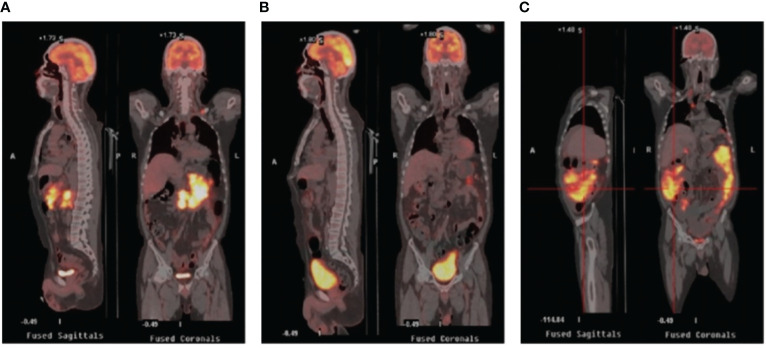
PET/CT images in the periods before CAR-T therapy and after CAR-T therapy. (A) Relapse after ASCT showing multiple enlarged lymph nodes with increased glucose metabolism. (B) PET/CT after combined targeted therapy showing fewer lesions with smaller volume and reduced metabolism. (C) PET/CT after CAR-T infusion showing rapid progression revealing increased lesions and metabolism. PET-CT stands for positron emission tomography-computed tomography; ASCT stands for autologous stem cell transplantation; CAR-T stands for chimeric antigen receptor T-cell.
